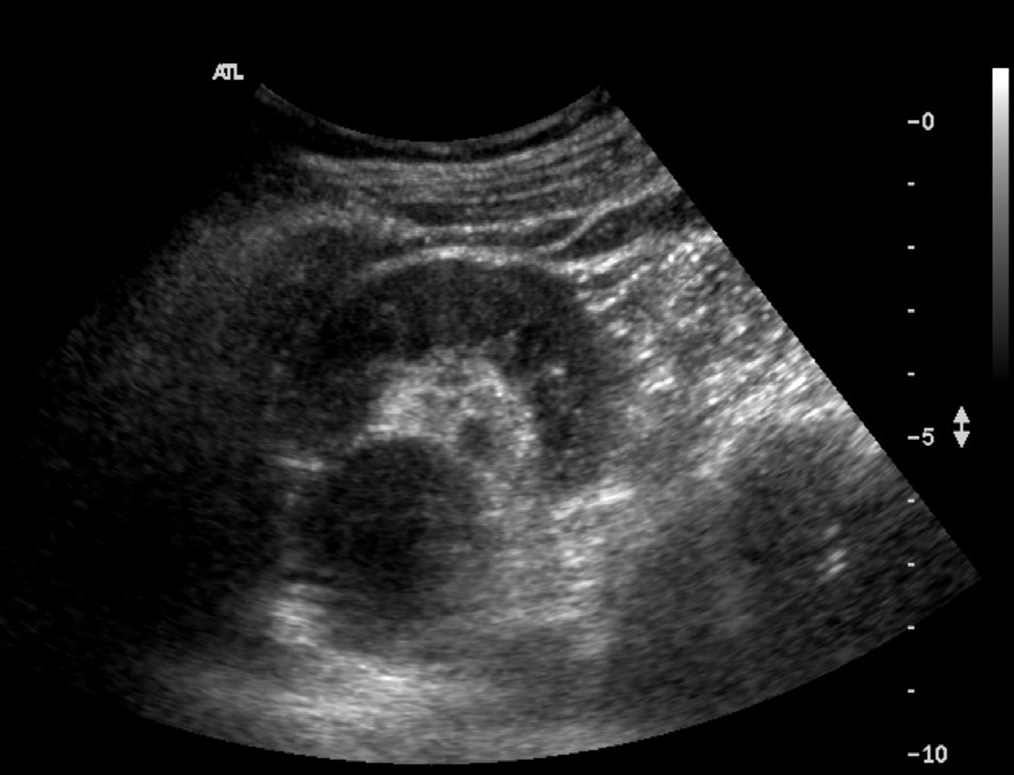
Ultrasound study of the left kidney during routine health check. Ultrasound
